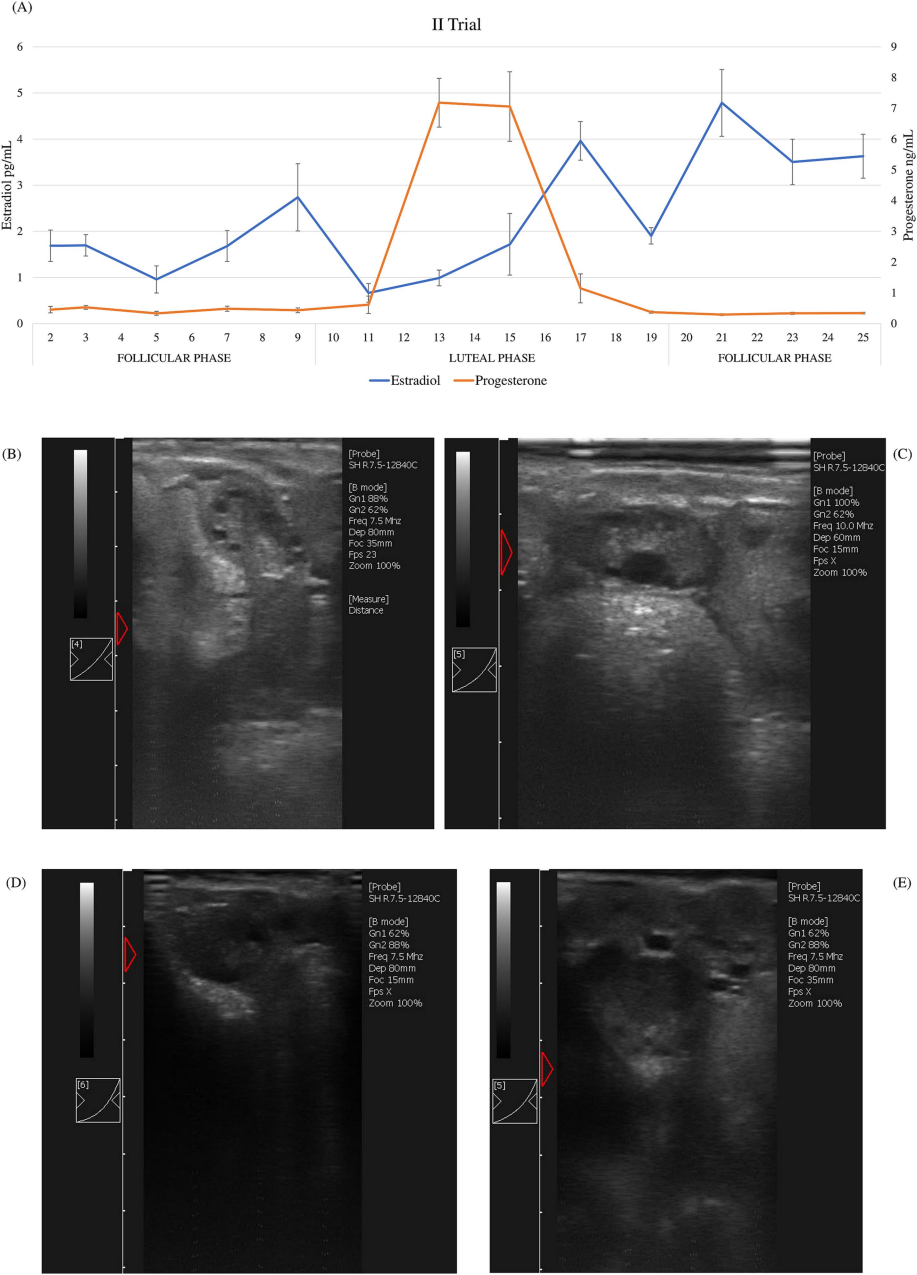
Second trial: (A) average of estradiol and progesterone concentrations (±SD) of ewes (*n* = 20) and ultrasound scan images of a representative ewe: (B) growing follicles at day 2; (C) dominant follicle at day 9; (D) early corpus luteum at day 11; (E) corpus luteum at day 15.
